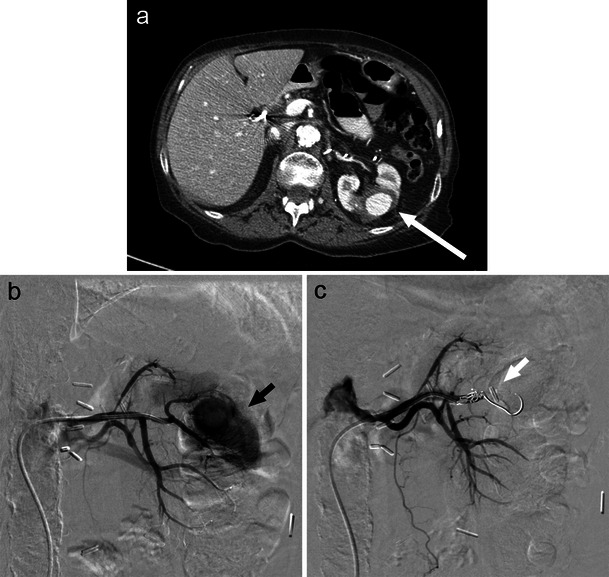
Axial CT (a, long white arrow) and angiographic (b, black arrow) images of a left intraparenchymal renal artery pseudoaneurysm subsequent to partial nephrectomy for renal tumour. The propensity for severe haemorrhage with these lesions warranted selective coil embolisation (c, white arrow)
